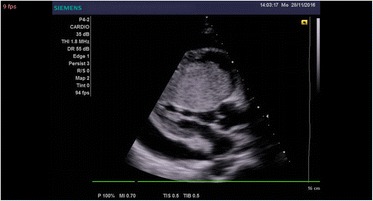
Transthoracic echocardiography showing right ventricular mass and pericardial effusion
